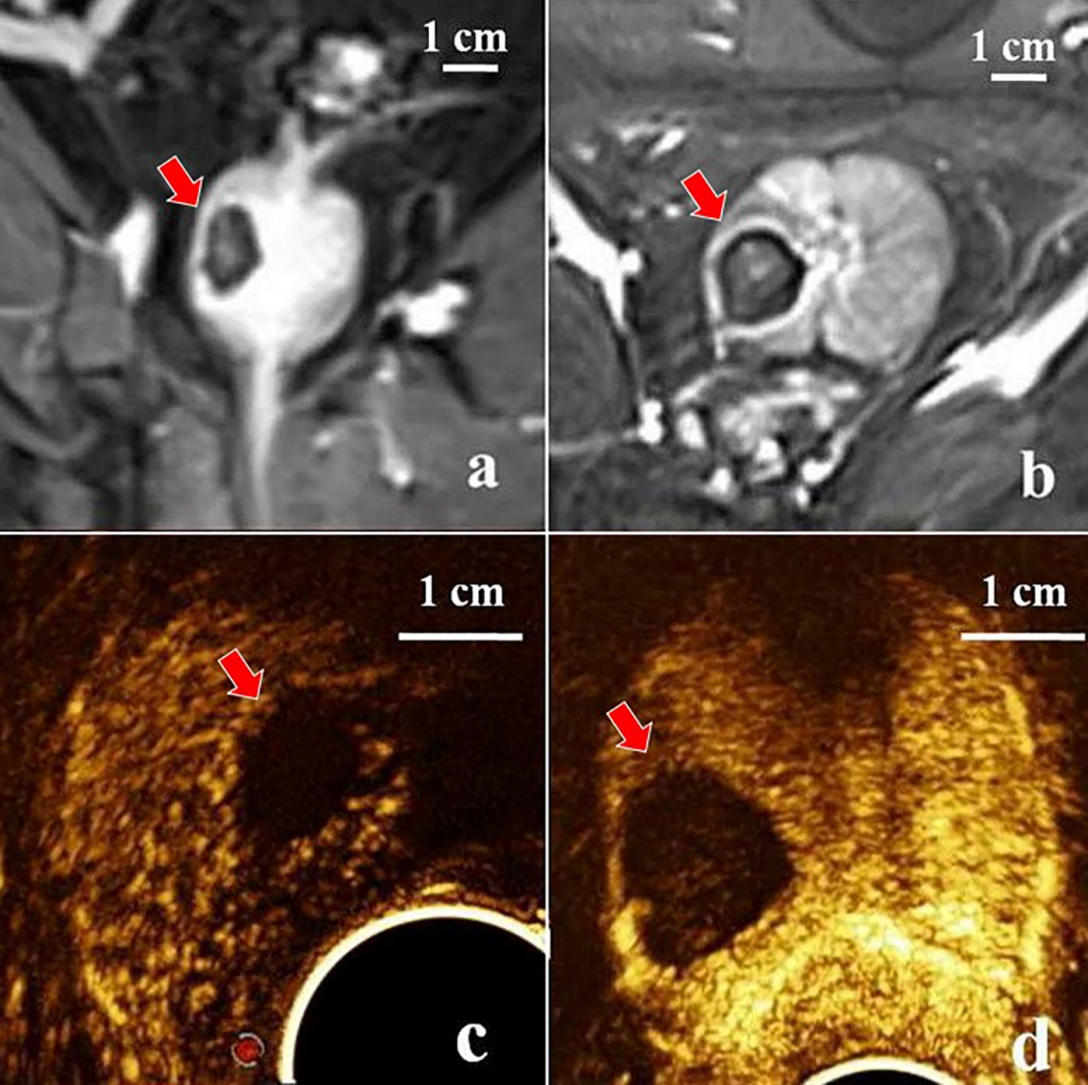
Comparison of the ablation zone in *in vivo* experiments. (a, b) Contrast-enhanced MRI imaging of the prostate after dual-fiber laser ablation. The ablation zone shows no filling of contrast agents (red arrow) and a weak signal; longitudinal (a) and transverse (b) sections are shown respectively; (c, d) CEUS imaging of the prostate after dual-fiber laser ablation. The ablation zone shows no filling of contrast agents (red arrow) and a weak signal; longitudinal (c) and transverse (d) sections are shown respectively.
